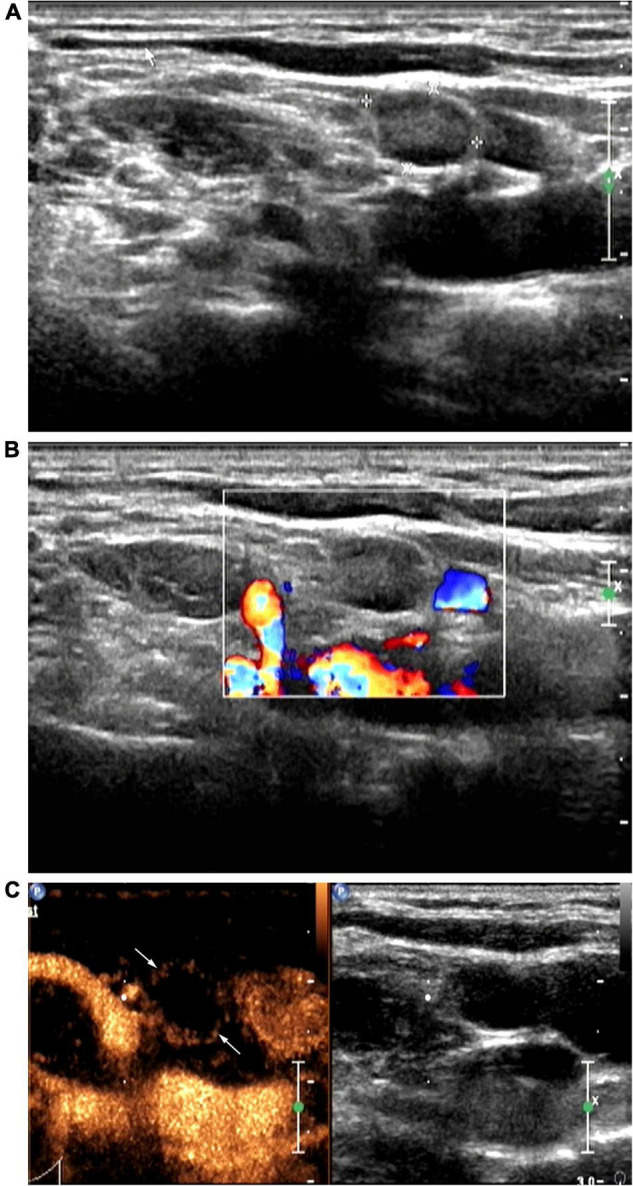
Patient with right cervical tuberculous lymphadenitis (CTL) (28-year-old man). Conventional ultrasound (US) showed type II CTL, which was re-classified as type III after contrast-enhanced ultrasound (CEUS). US-guided fine-needle aspiration was performed to obtain a small amount of pus. Pathological analysis of this was consistent with the manifestations of type III CTL. (A) Two-dimensional US indicating a lymph node (size, 1.0 × 0.7 cm) with a clear border, thickened cortex, uneven internal echoes, the hilum of lymph node was unclear. (B) No color blood flow signals. (C) Under peak CEUS enhancement, annular enhancement of the lymph node was observed, and a large area within the lymph node showed no enhancement.
